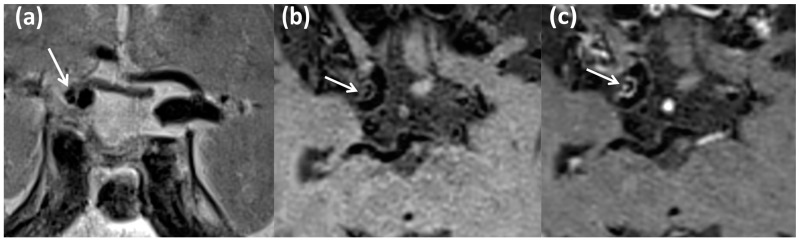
Patient ID#1. The MRI data correspond to stenosis of the left internal carotid artery (60–65%) with signs of an eccentric pattern of contrast enhancement. MRI in T2 mode with high resolution (T2-HR) in the coronary plane (a), MRI T1 db f/s, before (b) and after (c) intravenous contrast injection with high-spatial-resolution T1-weighed imaging and suppression of the signal from bloodstream and fat. White arrows—intracranial atherosclerotic plaque.
